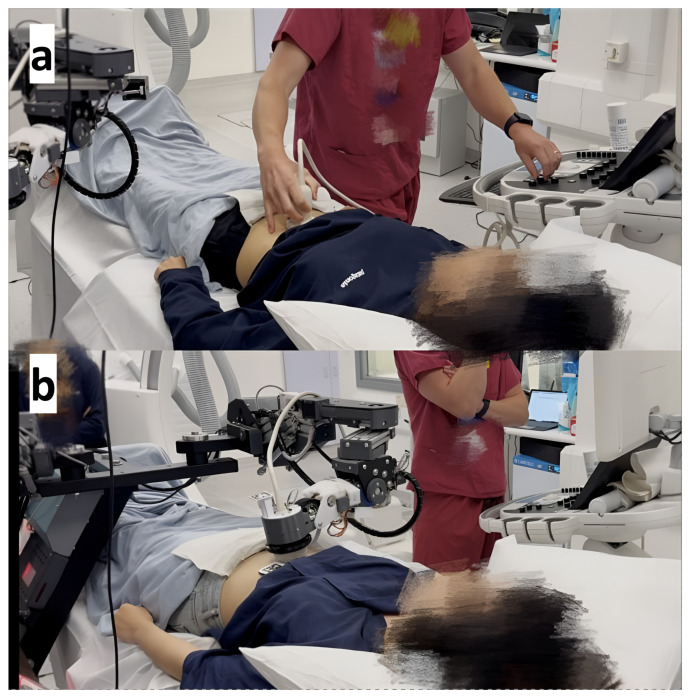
Stages of volunteer abdominal aorta ultrasound scanning protocol. (a) Manual reference scan performed by a trained clinician to localise the abdominal aorta and record probe force. (b) Robotic scan performed on the same volunteer using the autonomous system with integrated force control and patient-to-robot registration, under operator supervision.
